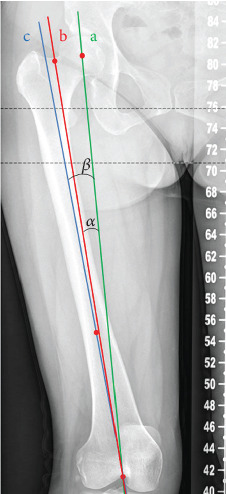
The upper VCA and the lower VCA on X-ray. Line a: femoral mechanical axis. Line b: femoral anatomical axis. Line c: distal femur anatomical axis. ∠*α*: the upper VCA. ∠*β*: the lower VCA.
